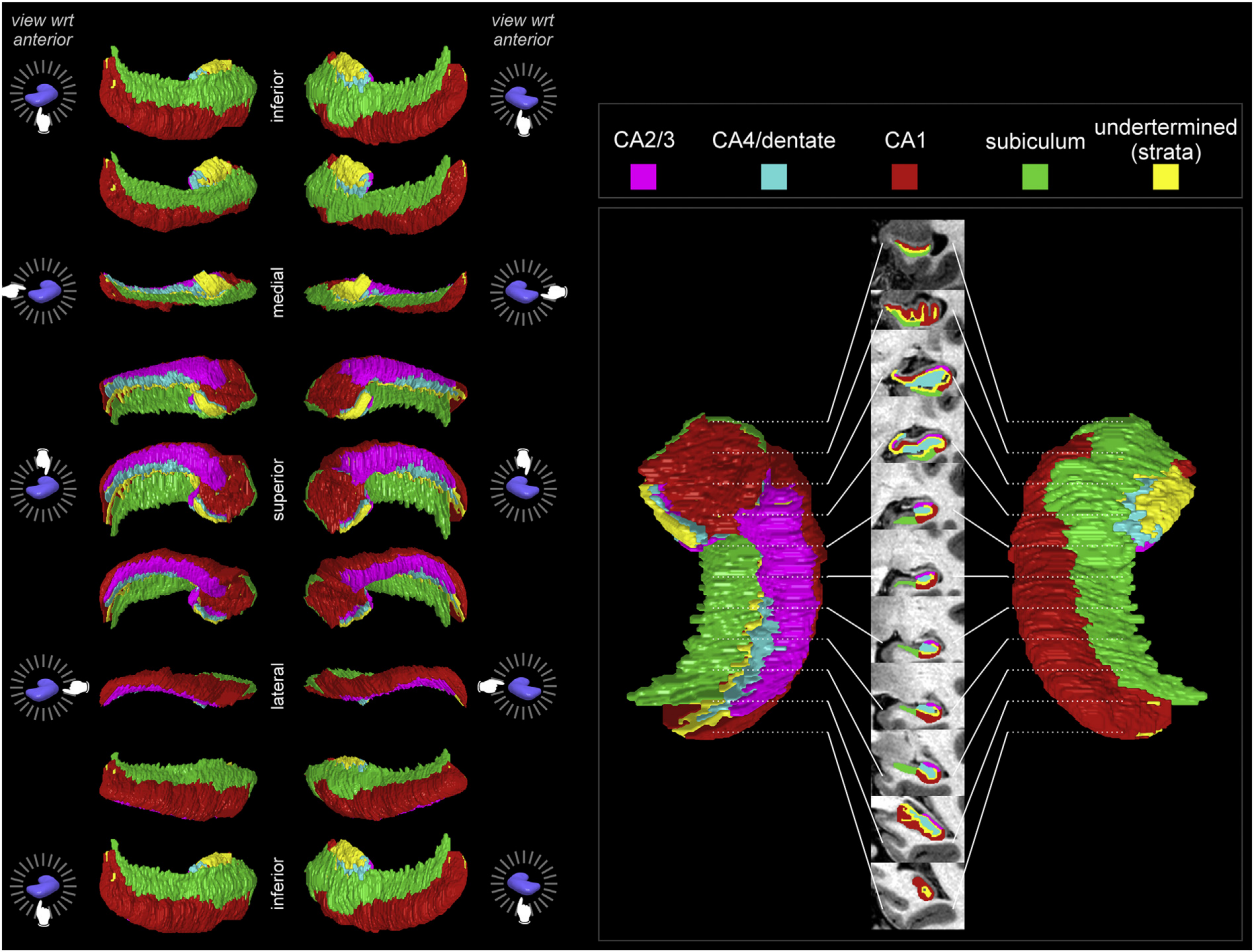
Hippocampal subfields based on the Winterburn atlas [subject 1; 37]. Left panel shows 45° rotations around anterior-posterior axis for surfaces of left and right hippocampi. Right panel shows right hippocampus dorsal (left) and ventral (right) representations, and the center shows coronal slices along anterior-posterior axis with the subregions overlaid onto the subject's high-resolution T1-weighted anatomical scan included with the Winderburn atlas.
